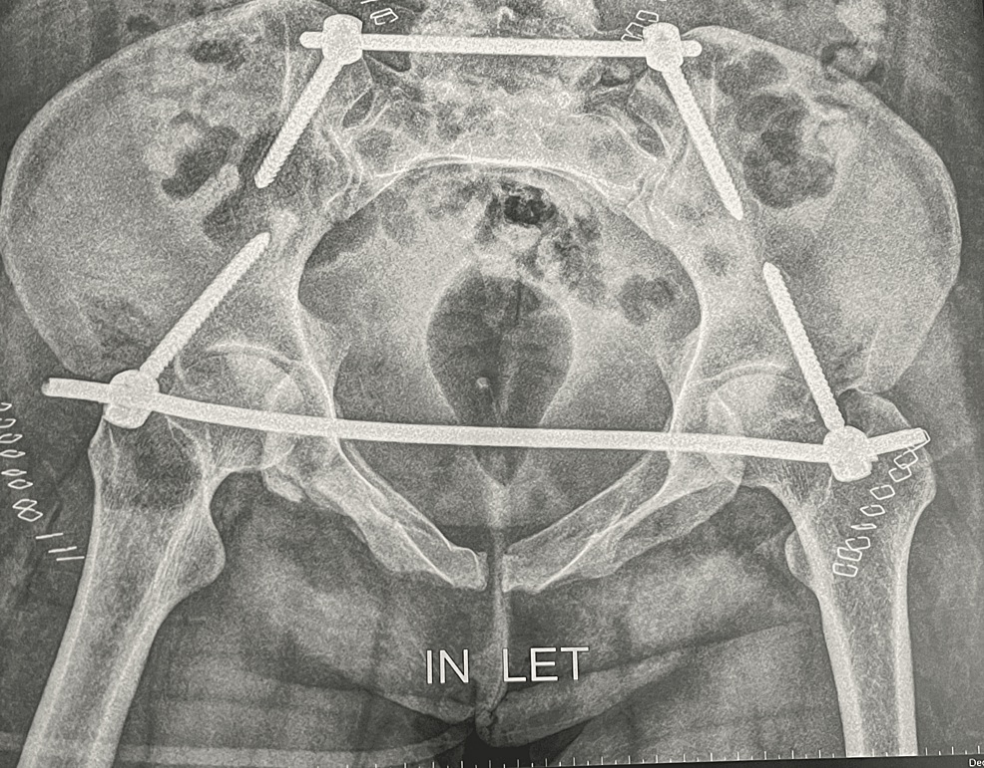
Postoperative X-ray inlet view of the pelvis showing excellent reduction of the sacral fracture with ilioilial fixator and good fixation of the anterior pelvic ring with INFIX with the overall good restoration of ring alignment Image Credit: First Author
